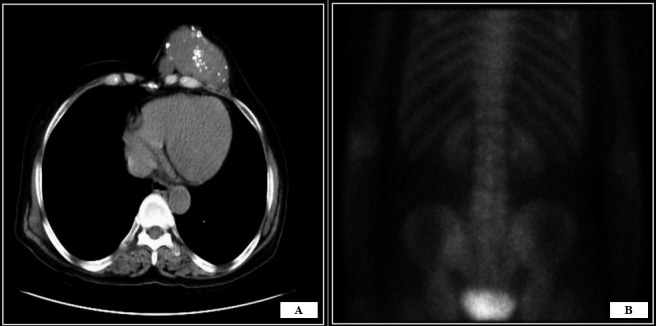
Figure 1. Imaging Findings of Breast Chondrosarcoma (A). CT thorax shows an ill-defined, minimally heterogeneously enhancing mass involving the upper inner quadrant of the left breast, measuring approximately 8.3 x 5.7 cm. It involves the underlying chest wall muscles. However, underlying rib is unremarkable, and (B). 99m-TC-MDP Bone Scan shows no scintigraphic evidence of skeletal metastases.
